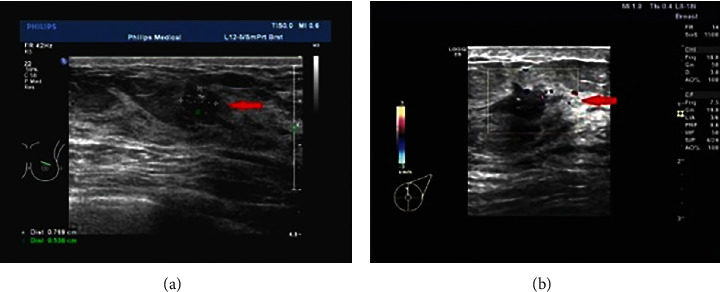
CDUS images of BC. (a) Two-dimensional US images. The arrow showed a two-dimensional US image showing a single nodule in the left breast, about 0.77 × 0.54 cm in size, with irregular shapes, fuzzy nodule edges, visible burrs, uneven internal echo, low echo, and spotting echo. (b) Color Doppler flow image. Arrows indicated abundant blood flow signals around the nodule. Color Doppler diagnosis was B1-RADS 5.
